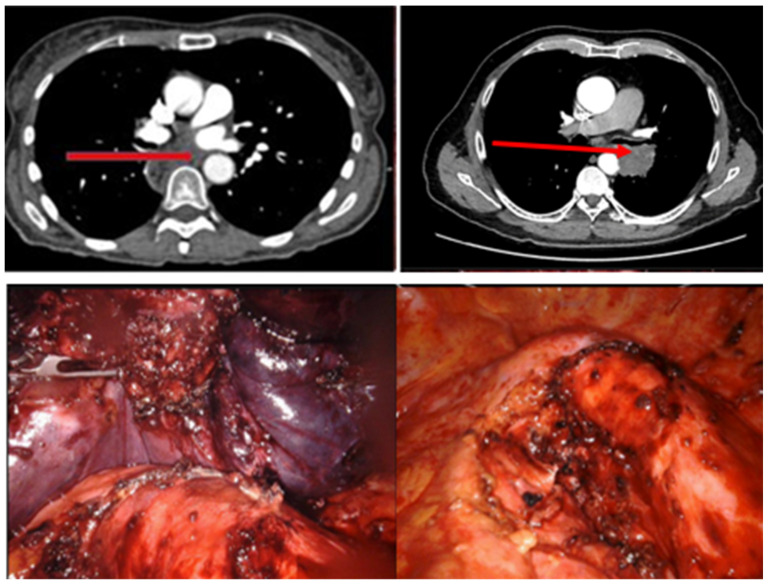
Top: Preoperative CT scan with the tumor mass in the posterior mediastinum with suspected aortic wall infiltration. Bottom: Intraoperative view of the partially resected tumor, including aortic wall.
